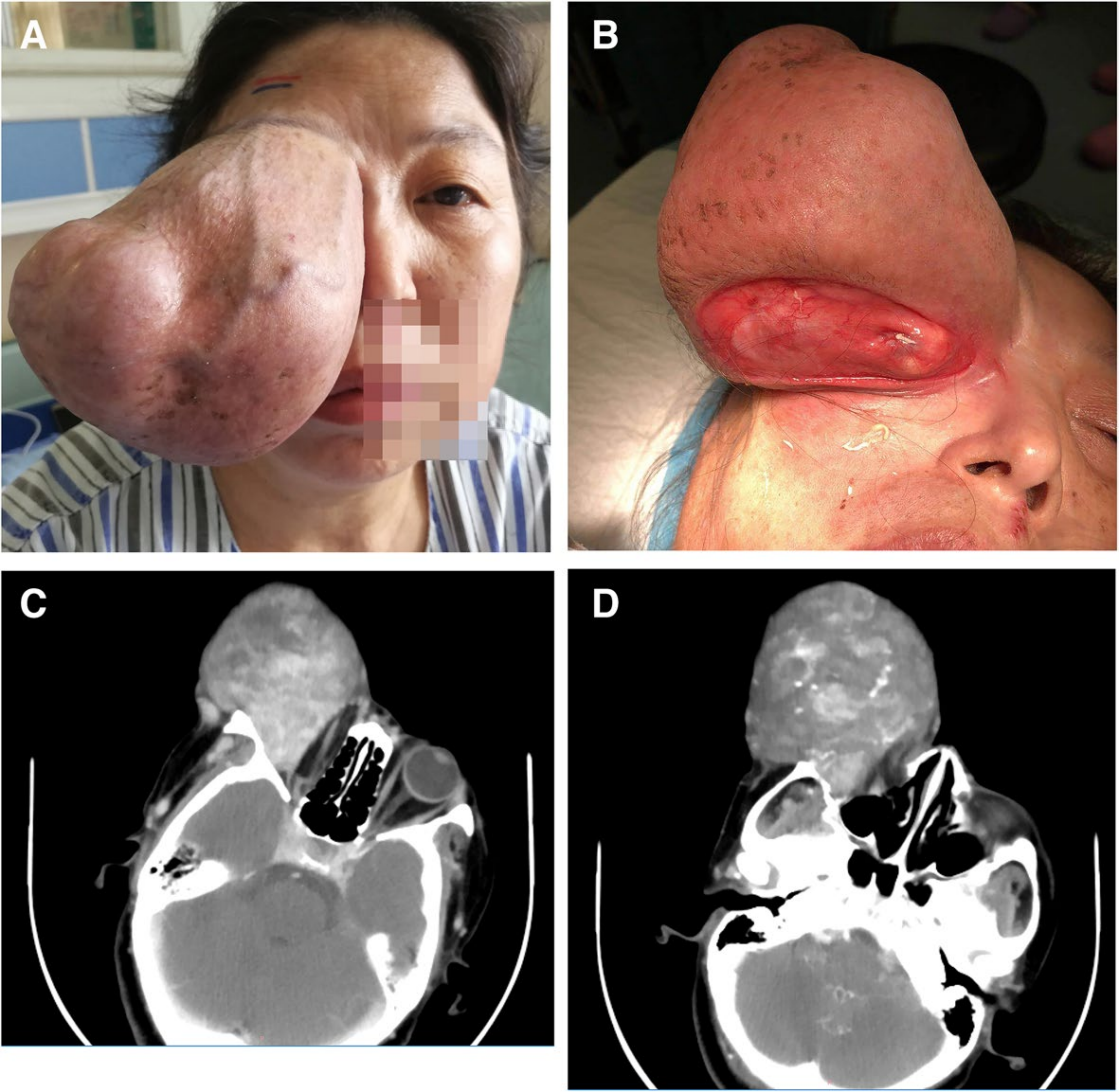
Preoperative photograph and findings of imaging studies. A A large multi-lobulated mass in the right orbit. B The palpebral fissure had widened, and the tumor had displaced the eyeball anteromedially (arrow). C Axial CT of the orbit shows a heterogeneous orbital mass. D Contrast-enhanced CT shows inhomogeneous and marked contrast enhancement of the mass. CT; computed tomography
